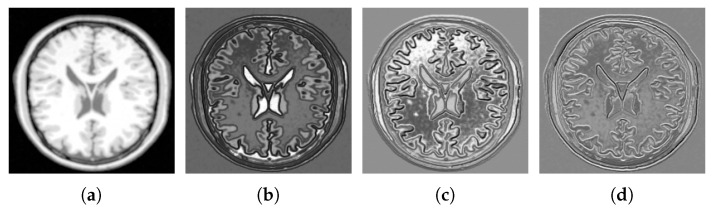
Structural representation of T1-MRI using Laplacian Eigenmap. Feature images indexed 1, 6, 11, and 18 are shown in (a–d), respectively.
